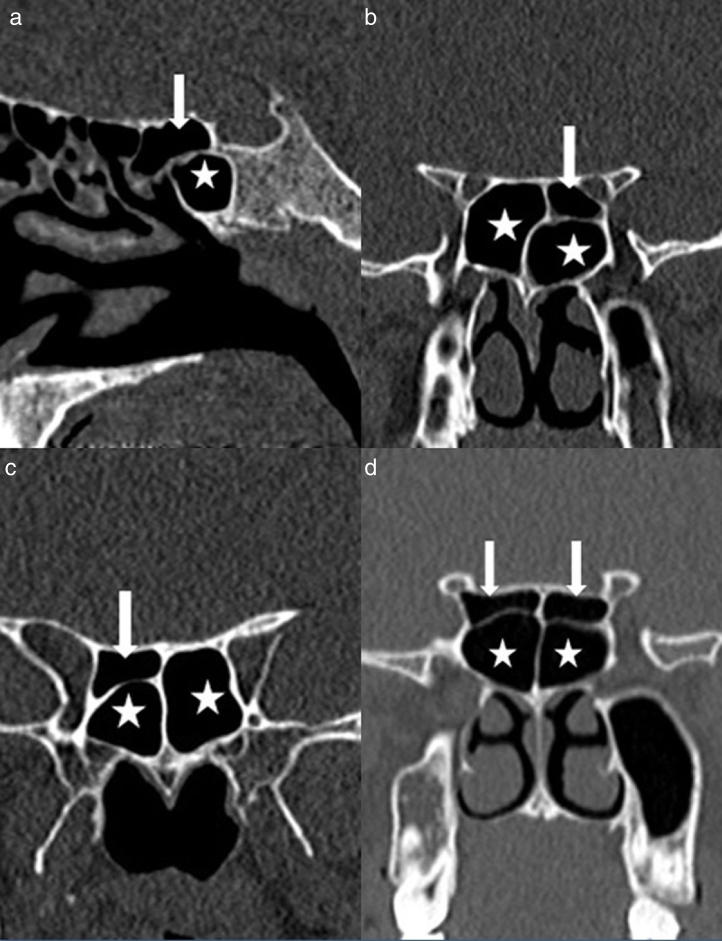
A coronal CT scan of the paranasal sinuses shows (a) sagittal image an Onodi cell; (b) coronal image of a left Onodi cell; (c) coronal image of a right Onodi cell; (d) coronal image of bilateral Onodi cells (arrow, Onodi cells; asterisk, sphenoid sinuses).
